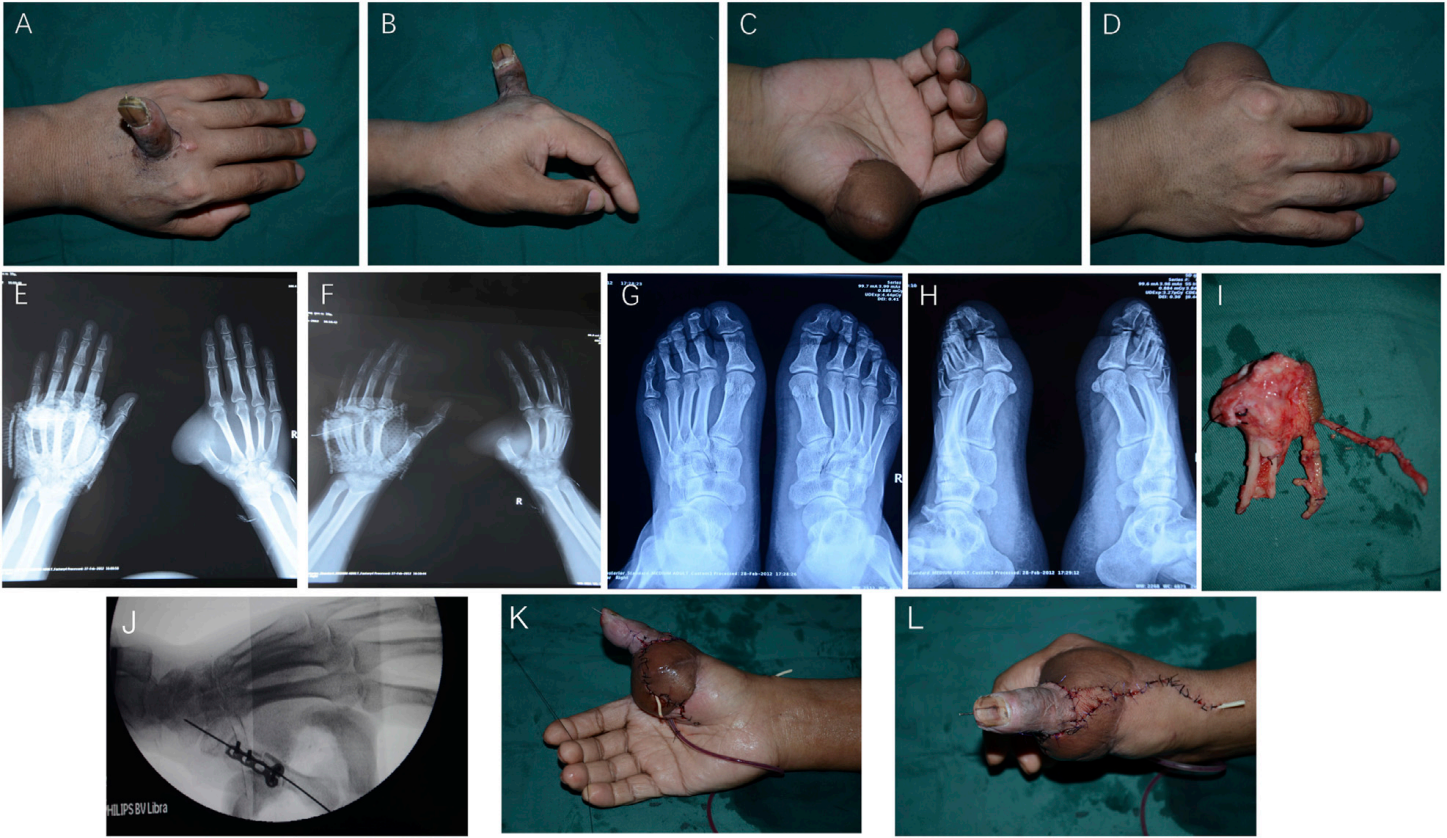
Thumb heterotopic replantation 5 months after the injury. (A, B) The wound was repaired by a groin flap. (C, D) X-rays of the hand and foot. (E–H) Free metatarsal phalangeal joint. (I) The metatarsal bone and the proximal end of the first metacarpal were fixed by a plate, and the phalange of the heterotopic replanted thumb was fixed by a Kirschner wire. (J–L) The color of the thumb and flap indicates good blood supply.
